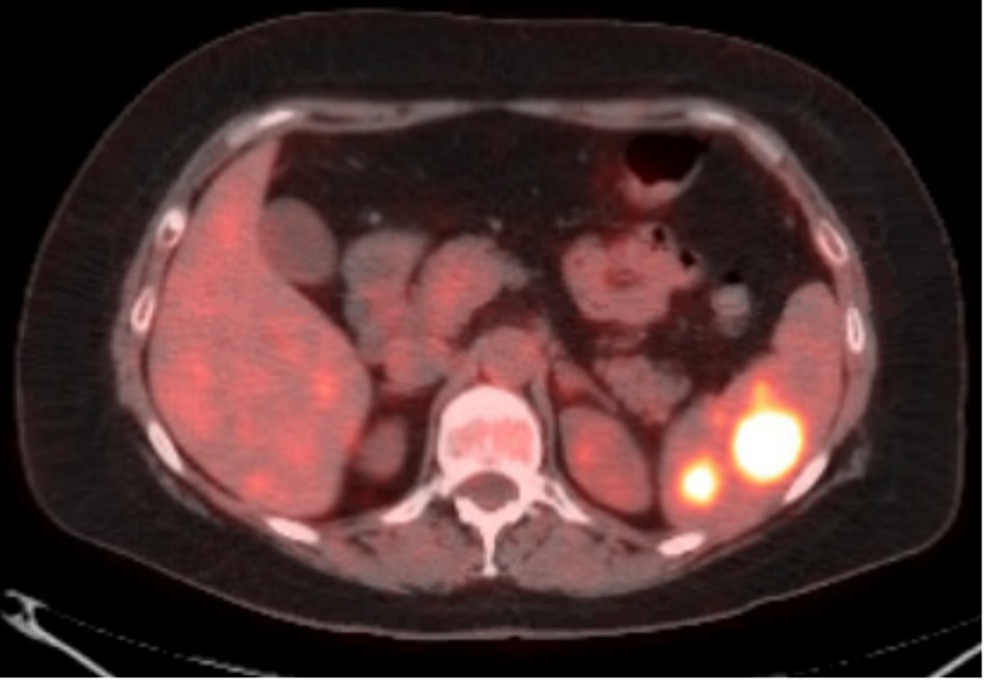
PET scan showing increased FDG uptake in the spleen PET: positron emission tomography; FDG: fluorodeoxyglucose
